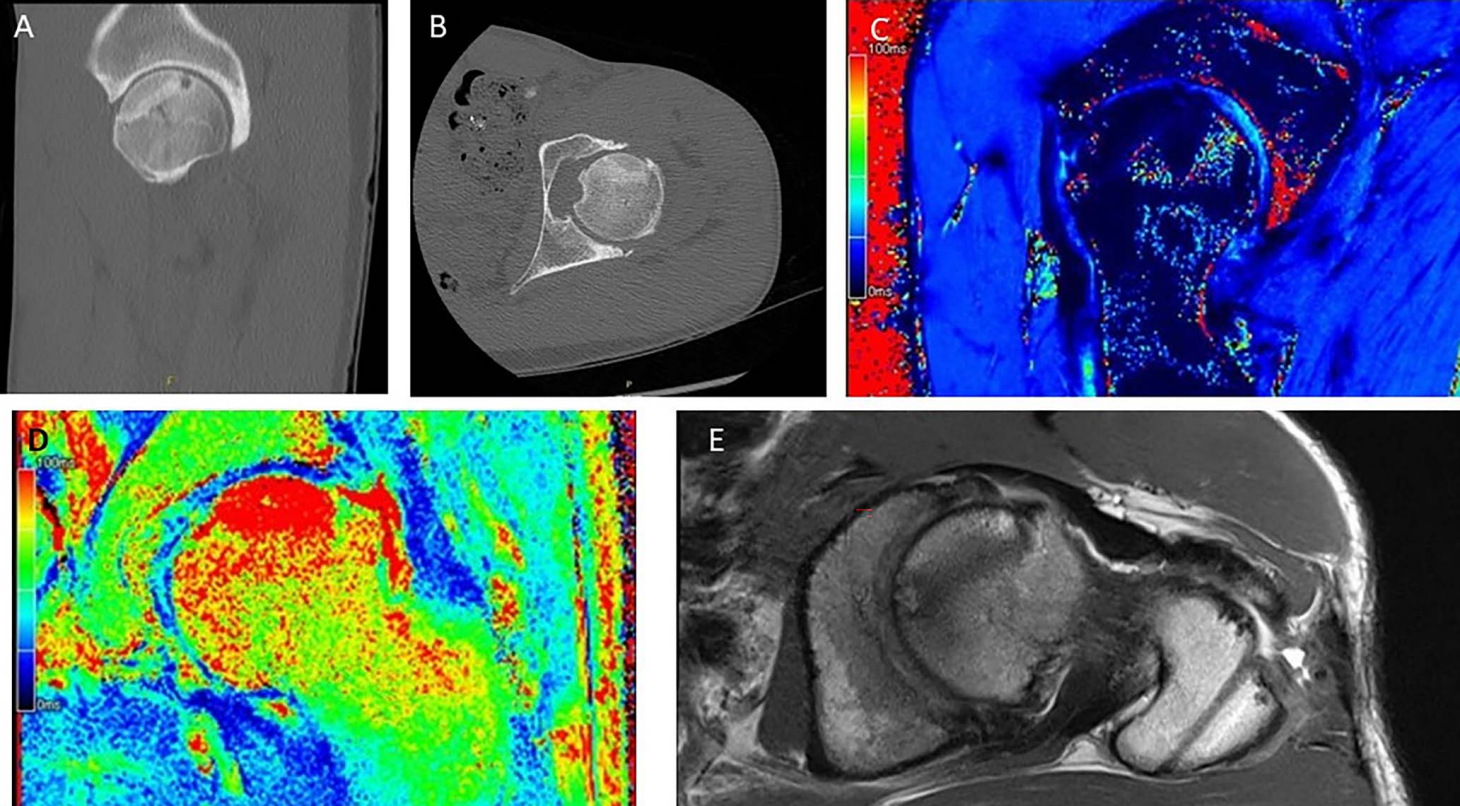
Postoperative imaging. (A) Computed tomography (CT) sagittal series demonstrates joint congruity and osseous integration of the left hip. (B) CT axial series demonstrates joint congruity and osseous integration of the left hip. (C and D) T2 magnetic resonance imaging (MRI) with cartilage mapping shows chondrocyte viability of the left hip. (E) MRI axial-oblique series demonstrates joint congruity and osseous integration of the left hip.
